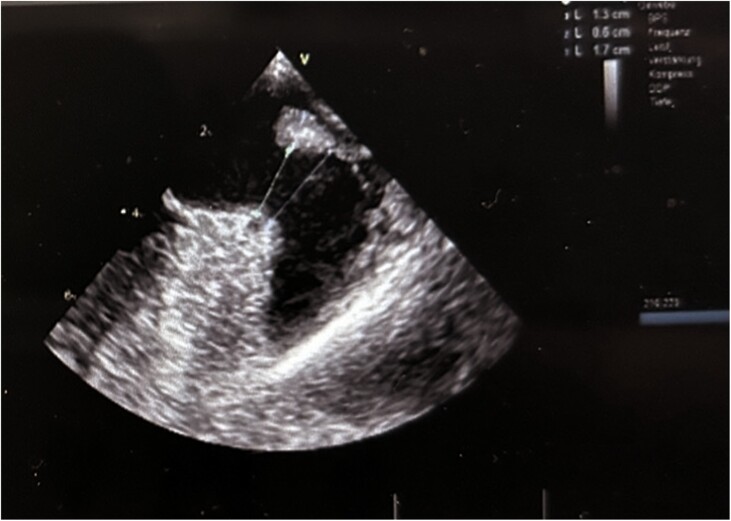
The maximal diameter of the left atrial appendage landing zone was 22.8 mm as measured on transesophageal echo.
